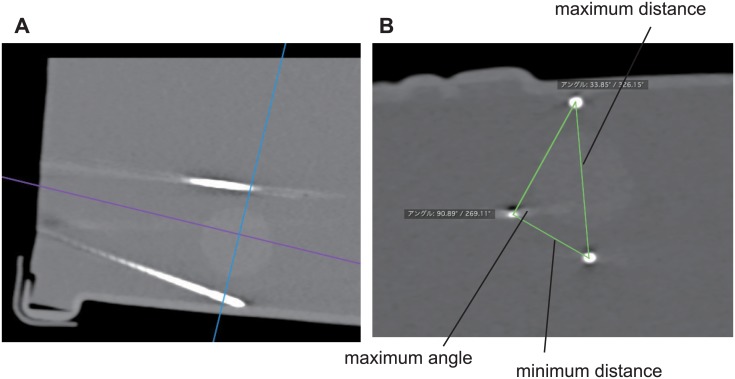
Definitions of angles and distance ratios. A) Computed tomography was performed immediately after the puncture procedure. B) The blue line represents the parasagittal plane. Each angle and distance between electrodes was measured.
